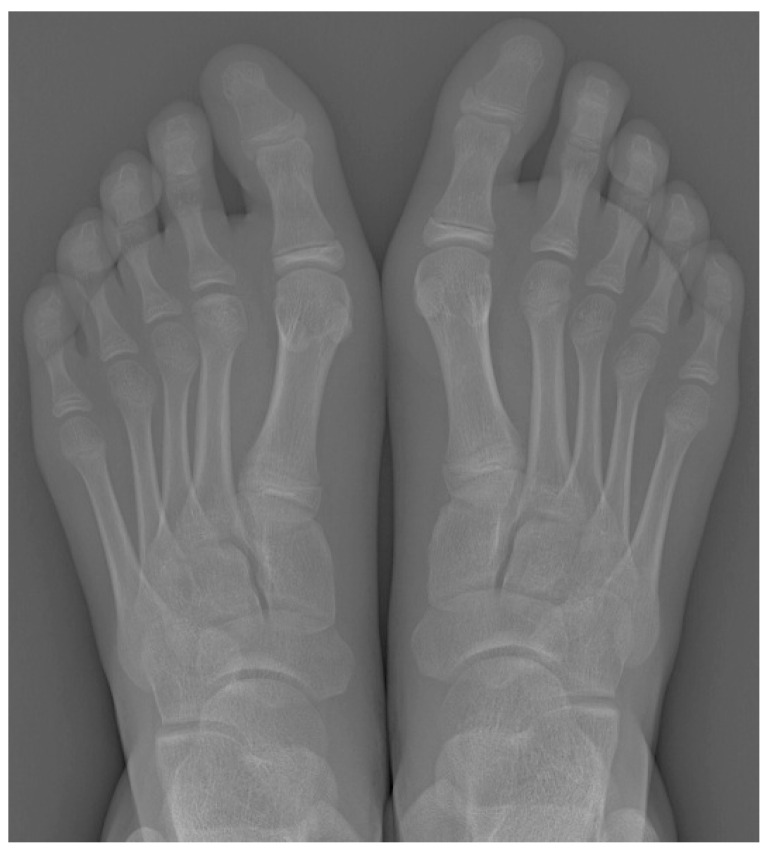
Radiograph of a symptomatic 10-year-old female patient showing early sclerosis and flattening of the second metatarsal head on the left foot, compared to the contralateral side.
